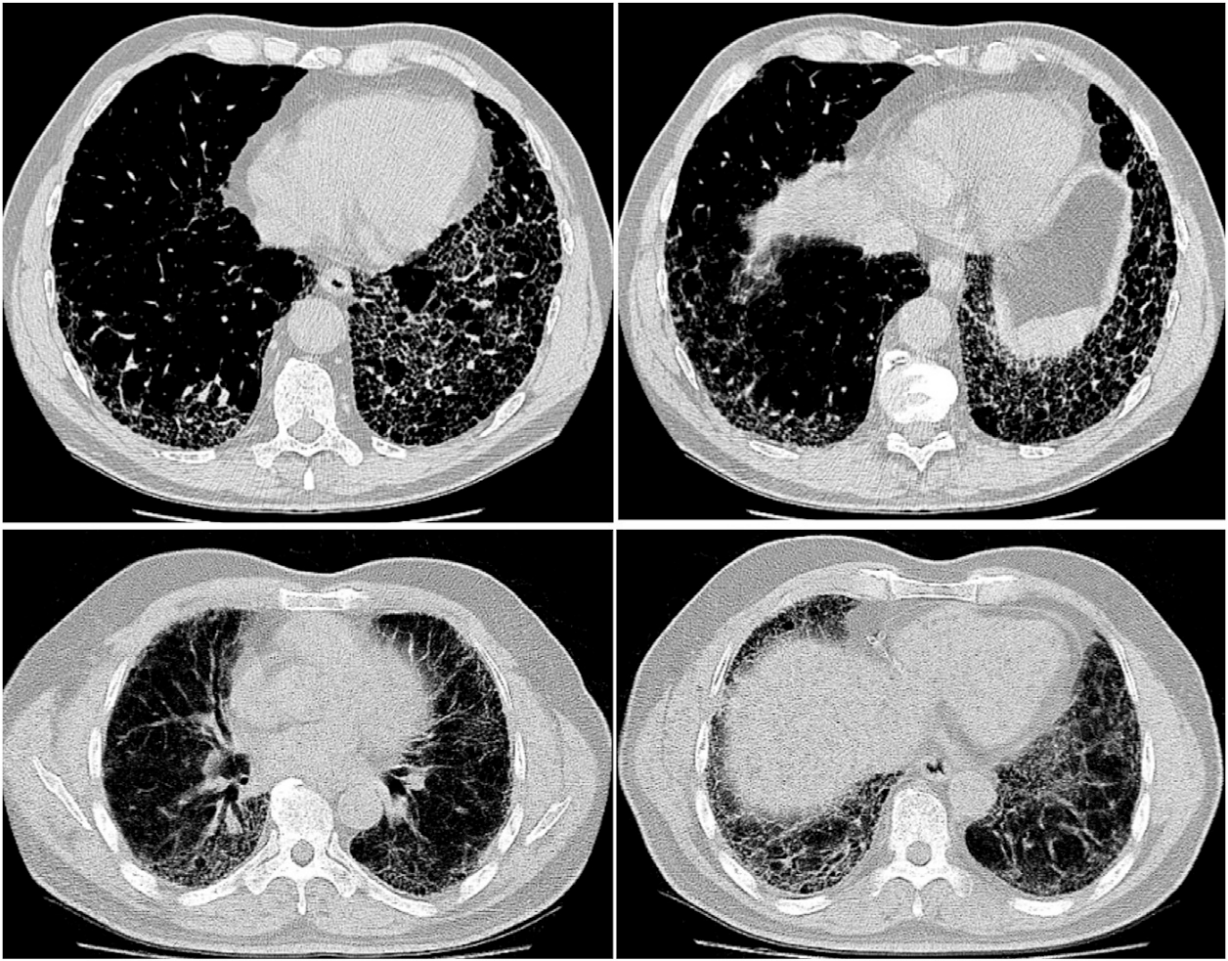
Example CT slices from the patient cohort from the OSIC Pulmonary Fibrosis Progression Challenge (OSIC, 2020).
